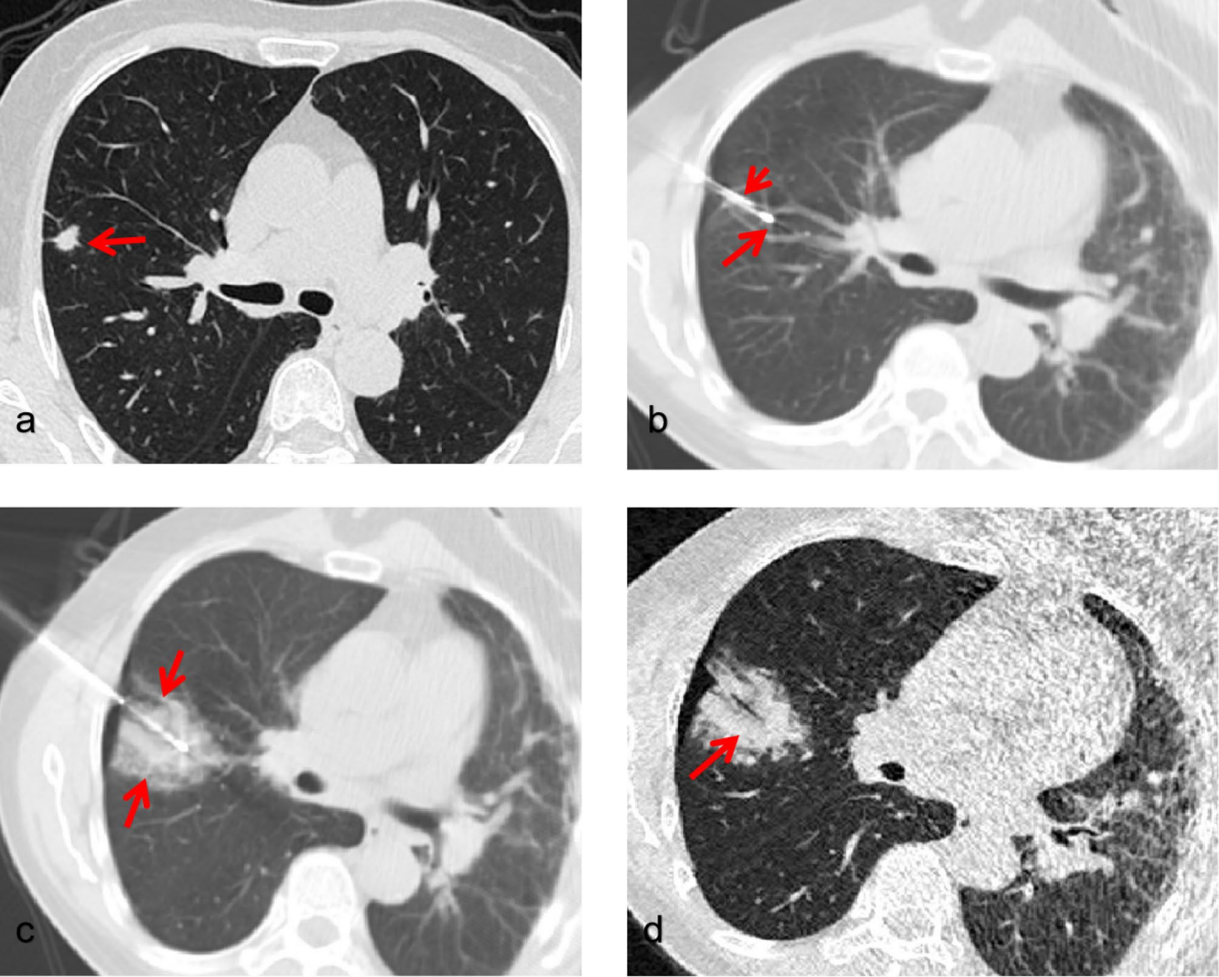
Synchronous procedure in a 77-year old woman. (a) Computed tomography image showing a single solid nodule (arrow), 11 mm ×6 mm, in the apical segment of the right superior lobe, regular margin, with pleural tail sign. (b) A cryoablation probe (long arrow) was placed into the nodule while the biopsy needle was at the edge of the lesion (short arrow). (c) During the cyroablation procedure, the locus center began to occur liquefactive necrosis as well as the ice ball emerged (arrows). (d) In the thoracic computed tomography scan immediately after cryoablation, the ablated nodule presented with a circular ground-glass opacity (arrow). Eventually, the pathological result showed it a lung adenocarcinoma
